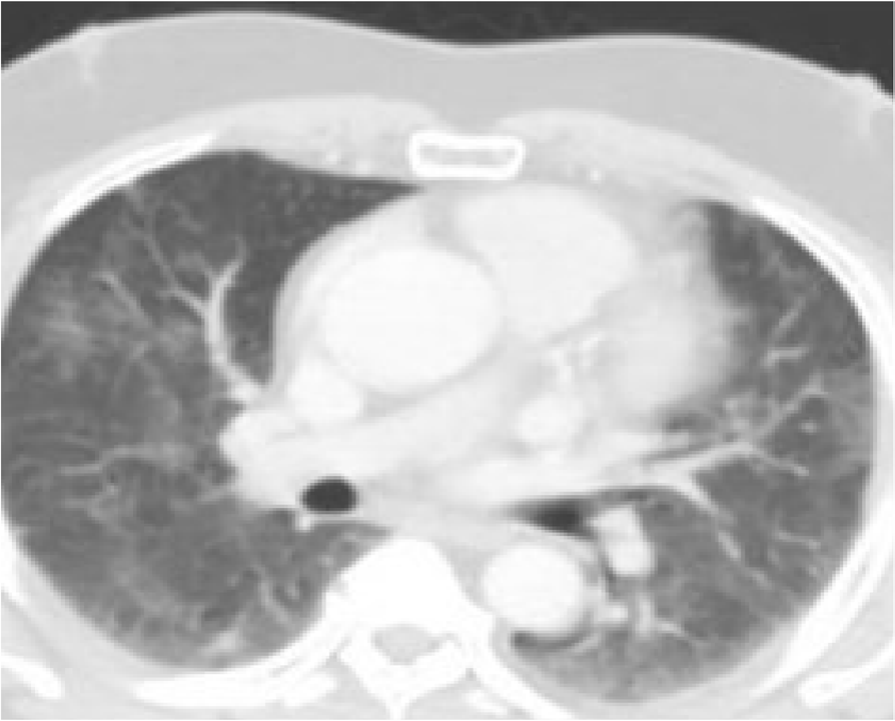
CT chest: bilateral multifocal patchy areas of ground glassing associated with reticulations and fibroelastic bands, probably post-COVID-19 pulmonary fibrosis (sequelae of post-COVID-19 viral infection)
